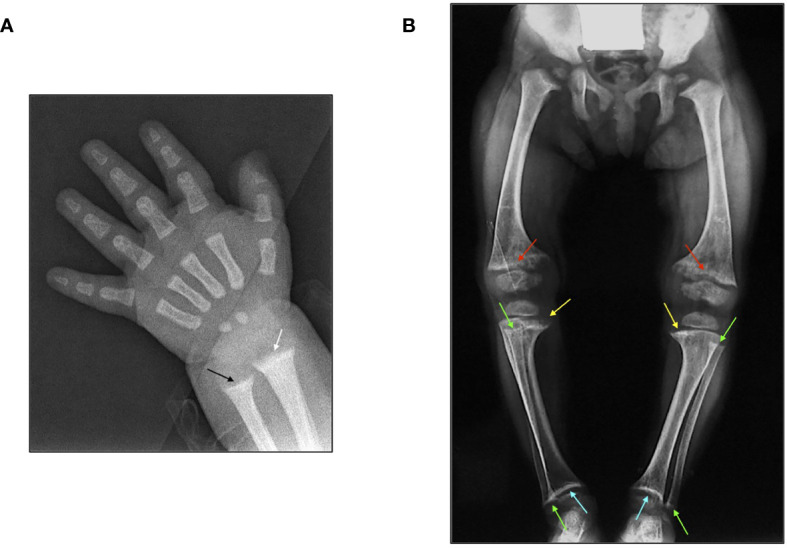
(A) X-ray features of the wrist in a 3-month-old male patient affected by XLH at diagnosis: widening and fraying of the epiphyseal plate (white arrow) and metaphyseal concavity of the ulna (black arrow). (B) X-ray features of the lower limbs in a 2.5-year-old female patient with XLH at diagnosis: genu-varum and distal medial femoral and tibial bowing with widening and fraying of the distal epiphyseal plate of the femur (red arrow) and the proximal medial epiphyseal plate of the tibia (yellow arrow). Metaphyseal concavity of the proximal and distal fibula (green arrow) and metaphyseal concavity of the distal epiphyseal plate of the tibia (light blue arrow).
